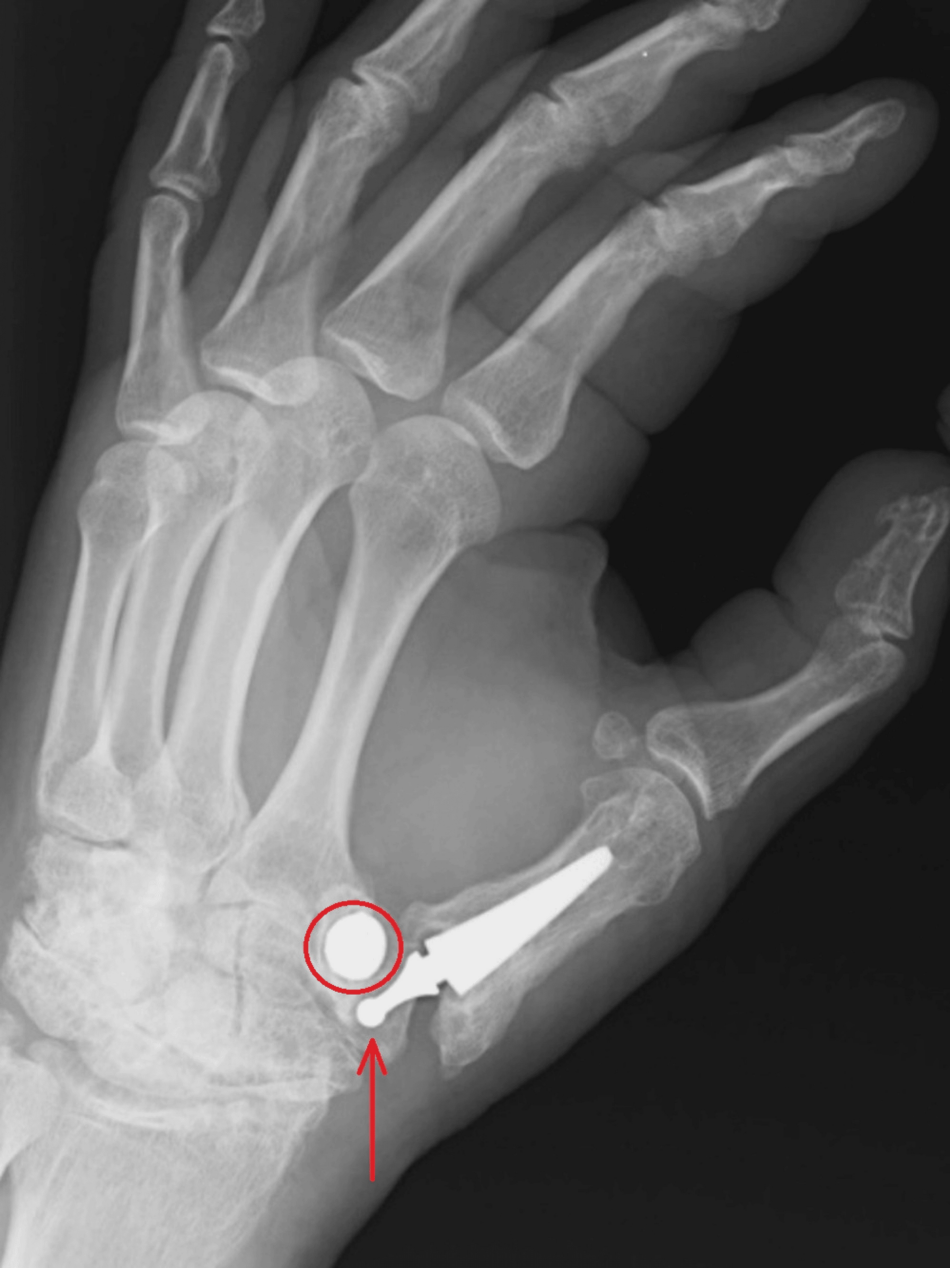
X-ray in Robert's projection obtained three years after surgery The figure shows dislocation of the implanted endoprosthesis elements. The red arrowhead marks the dislocated head of a double mobile CMC thumb prosthesis. The open circle marks the metal acetabular shell.
